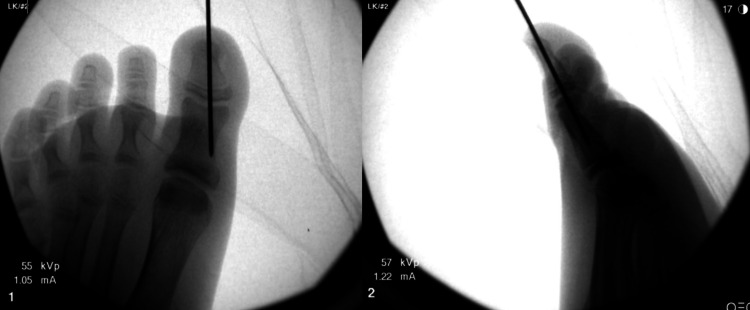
Intra-operative AP (left) and lateral (right) fluoroscopic radiographs of the left great toe demonstrating reduction and stabilization of a Salter-Harris I fracture of the distal phalanx with a Kirschner-wire. AP, anteroposterior.
